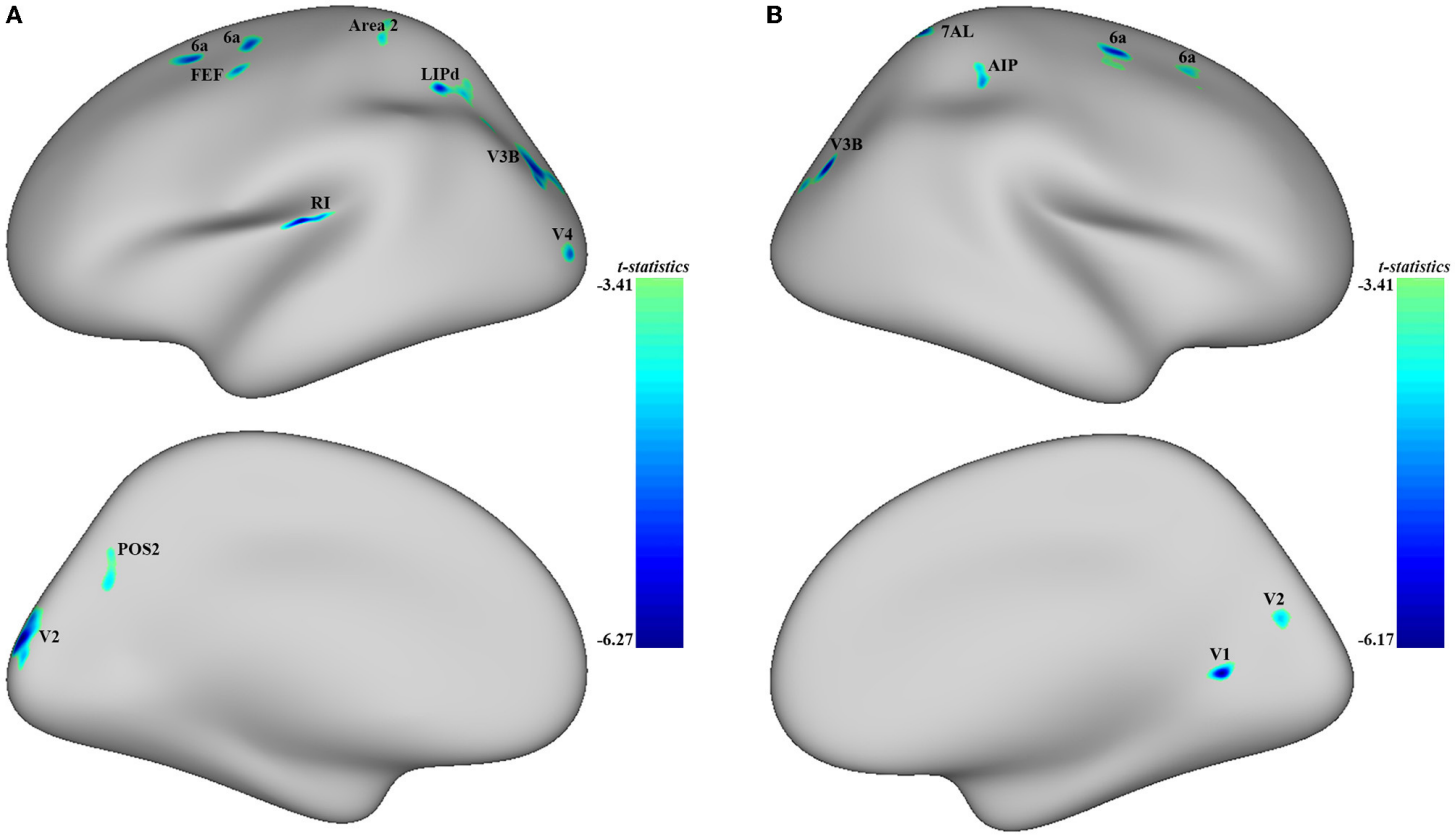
Adolescent MDD showed significantly decreased CT at both the left and right hemispheres. (A) Adolescent MDD showed significantly decreased CT value at several clusters including the LIPd (superior parietal cortex), V3B (dorsal stream visual cortex), V2 and V4 (early visual cortex), RI (early auditory areas), POS2 (posterior opercular cortex), and 6a and FEF (premotor cortex) at the left hemisphere. The color bar depicts the *t*-statistics; (B) Adolescent MDD showed a significantly decreased CT value at several clusters including the 6a (superior premotor subdivision), V3B (dorsal stream visual cortex), AIP and 7AL (superior parietal cortex), V1 (primary visual cortex), and V2 (early visual cortex) at the right hemisphere. The color bar depicts the t-statistics.
